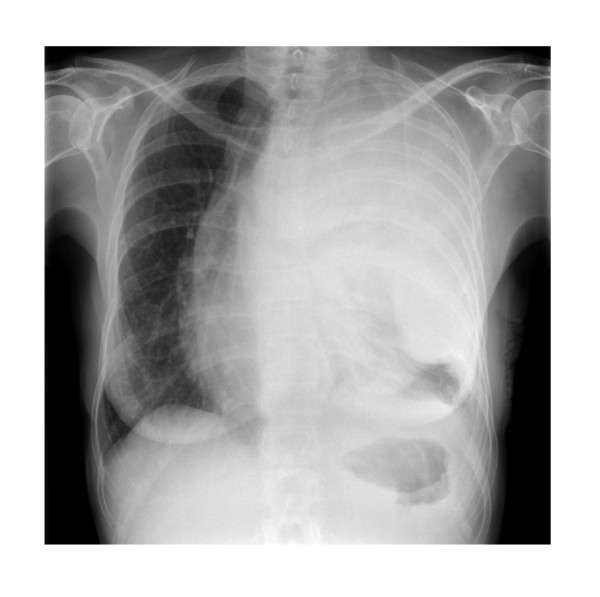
Chest Radiograph, November 2006.
